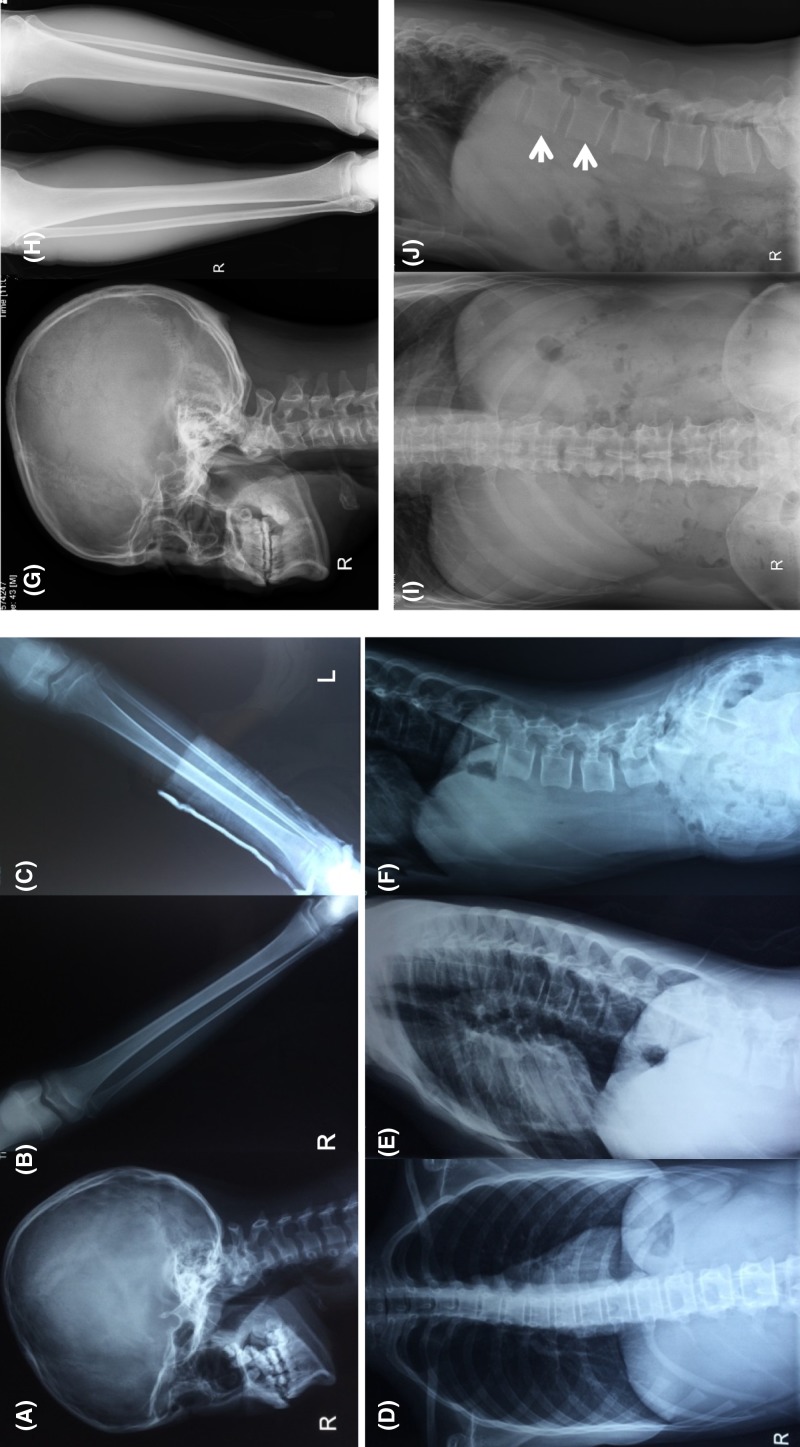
X-ray films of bone of the proband and her father (A–F) Imaging features of the proband; (A) no wormian
